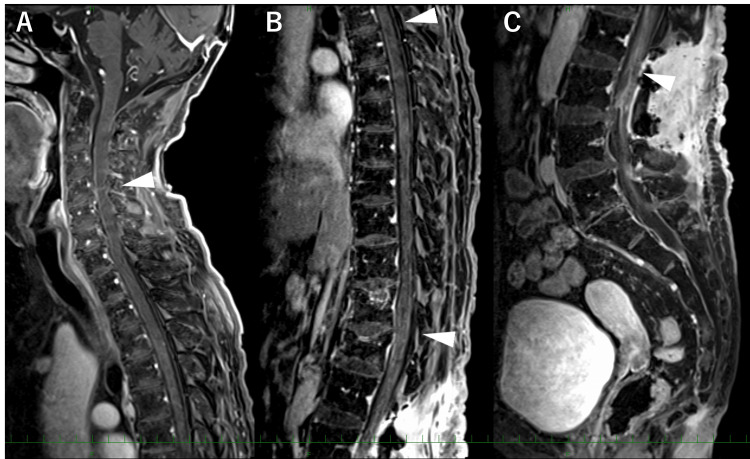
Images after treatment Whole spine Gd T1-weighted MRI shows tumor regression after radiation therapy.
